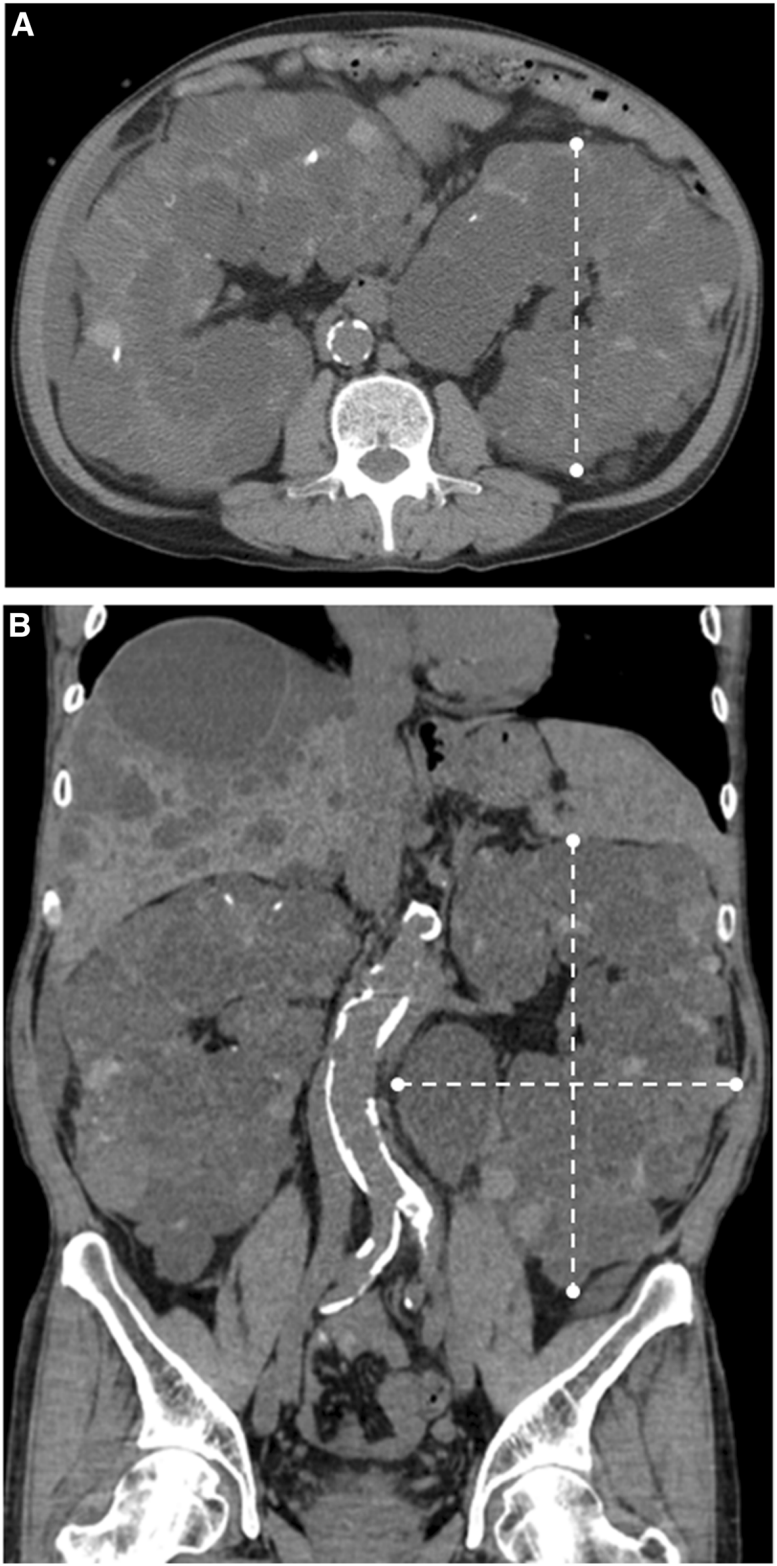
Computed tomography showing innumerable cysts of variable size in both kidneys and the liver. (A) Axial and (B) coronal unenhanced computed tomography showing enlarged kidneys and multiple liver cysts. The three greatest diameters of the kidney are indicated by dashed lines. No tumors were identified in other organs.
